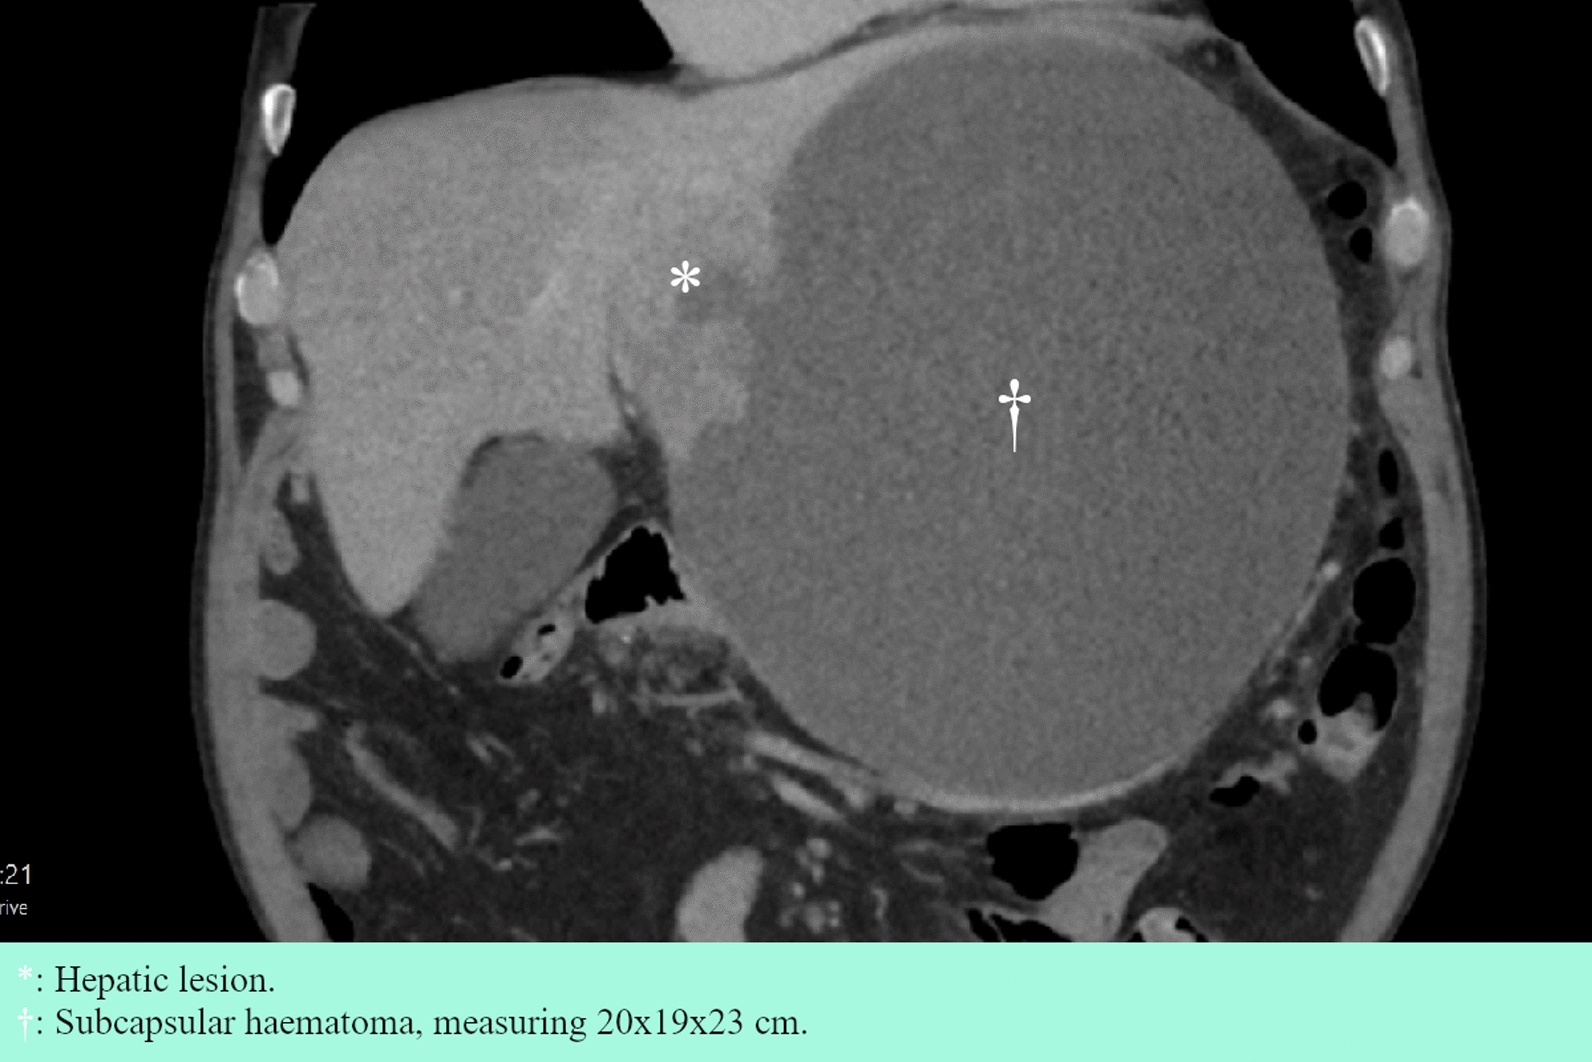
Coronal section of abdominal CT scan, 3 months after initial admission
